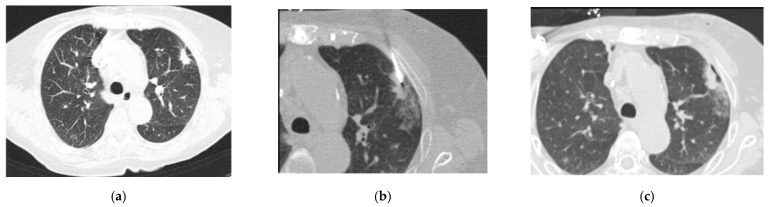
Axial CT images of the lungs conducted with the use of 90 mAs at 120 kV, and a 3 mm slice thickness with a 2 mm increment: (a) a pulmonary mass seen on the left, immediately before the biopsy; (b) the needle entering the pleura at an angle away from the perpendicular; and (c) a stable pneumothorax observed in the post-biopsy CT scan.
